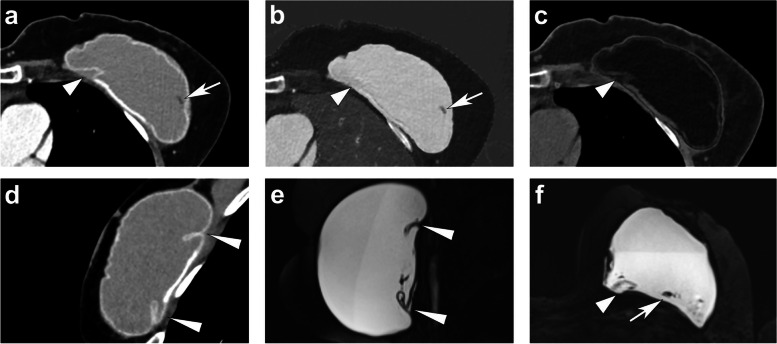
Photon-counting computed tomography images with monoenergetic reconstructions (a axial; d sagittal) and material decomposition reconstructions (axial iodine map [b] and virtual unenhanced [c]) as well as magnetic resonance imaging silicone-sensitive sequences (e sagittal; f axial) of a breast implant on the left side with intracapsular rupture. Peri-implant silicone collections (short thick arrowhead in a, b, c, f), keyhole sign (long thin arrowheads in d and e), and small intraimplant fluid collections (arrow in a, b, and f) can be detected on both modalities
